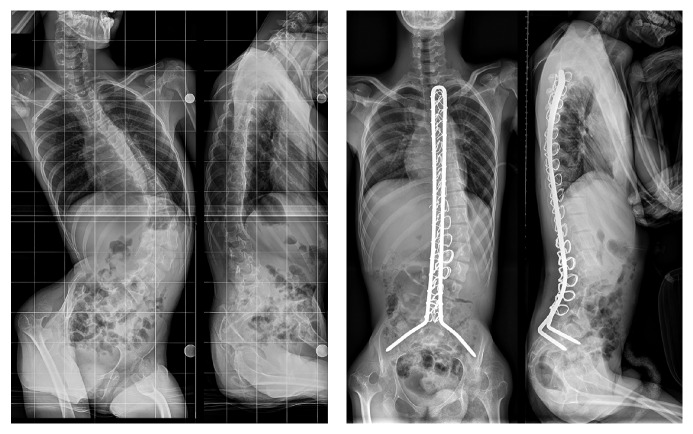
Pre- and postoperative radiography of long spinal fusion using Unit Rod technique in a patient with neuromuscular scoliosis due to cerebral palsy.
